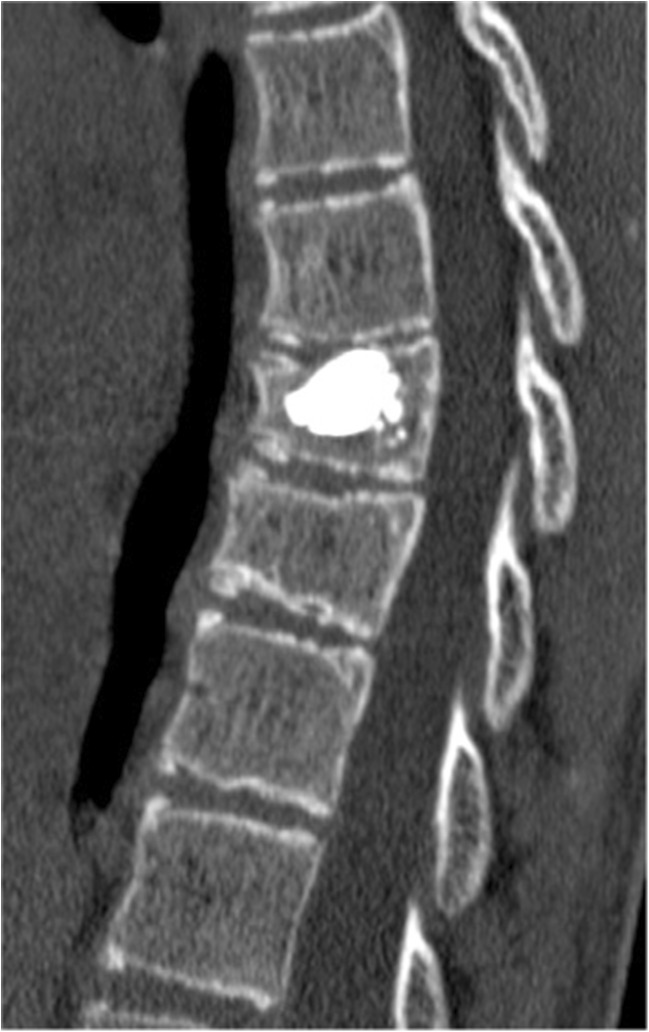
CT control scan of VB (sagittal)
